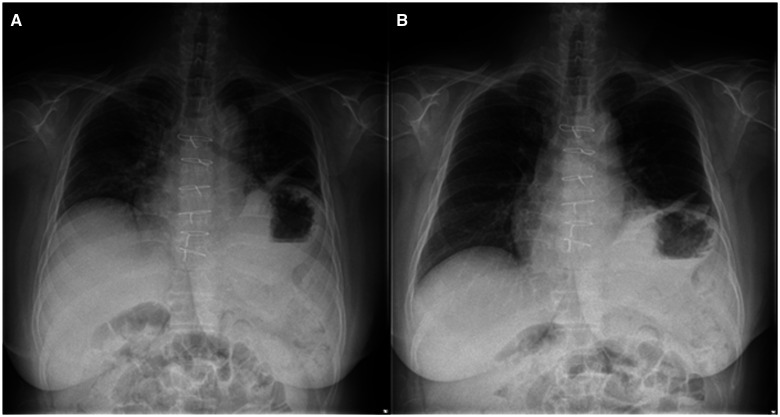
Preoperative chest radiograph. The left hemidiaphragm is markedly elevated and does not move with (A) exhalation and (B) inhalation, which shows downward movement of the right diaphragm.
